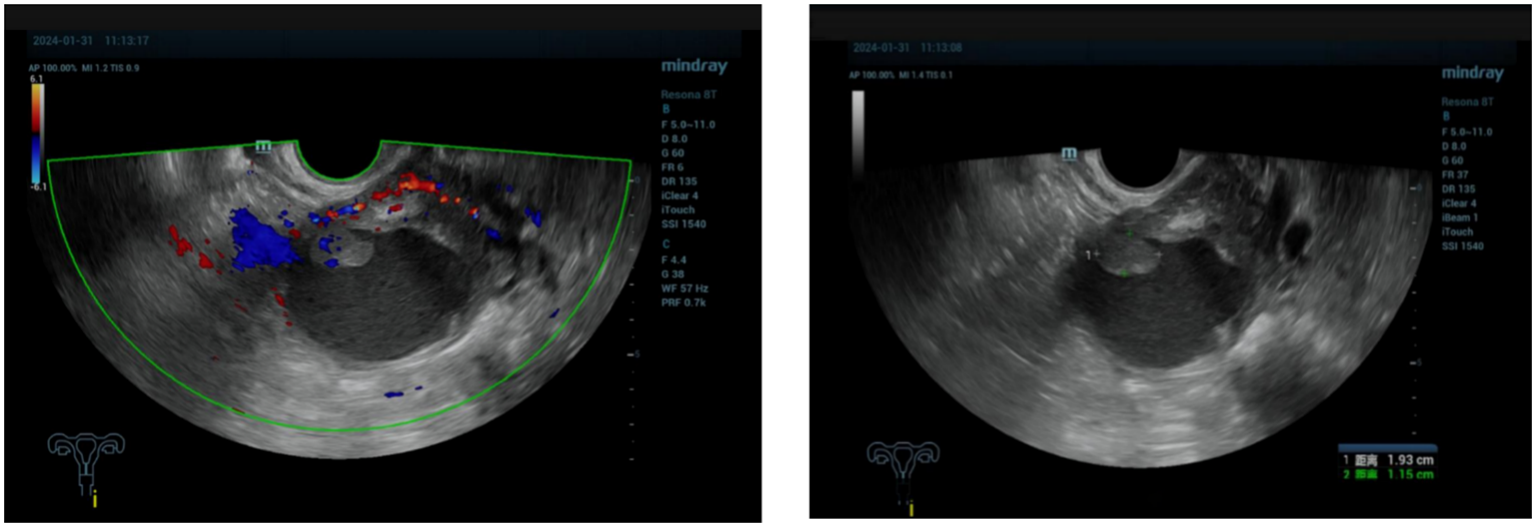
The ultrasound showed enhanced echo in the lower segment of the uterus, ranging from 5.6×4.8×3.8cm, considering endometrial malignancy.
